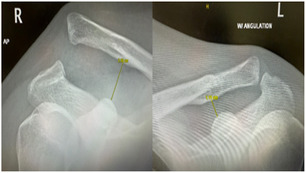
Upright anteroposterior radiographs comparing the affected and contralateral shoulders. The right shoulder shows increased coracoclavicular distance of 2.35 cm compared with 1.15 cm on the left shoulder, indicating marked acromioclavicular joint separation.
